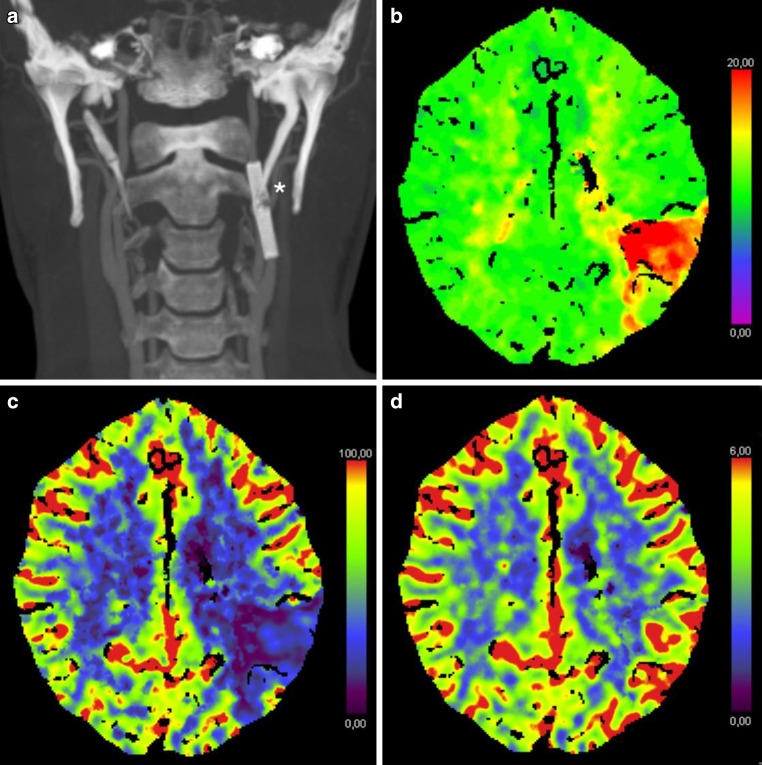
Coronal maximum intensity projection (MIP) of the CT angiography scan (a) depicts the fractured ICA stent in close vicinity to an elongated and thickend left styloid process (white asterix in a). Contrast enhanced CT perfusion study (b, c, d) reveals elevated time to peak (b), impaired cerebral blood flow (c), and preserved cerebral blood volume (d) of the left postcentral region in the posterior vessel territory of the left MCA
